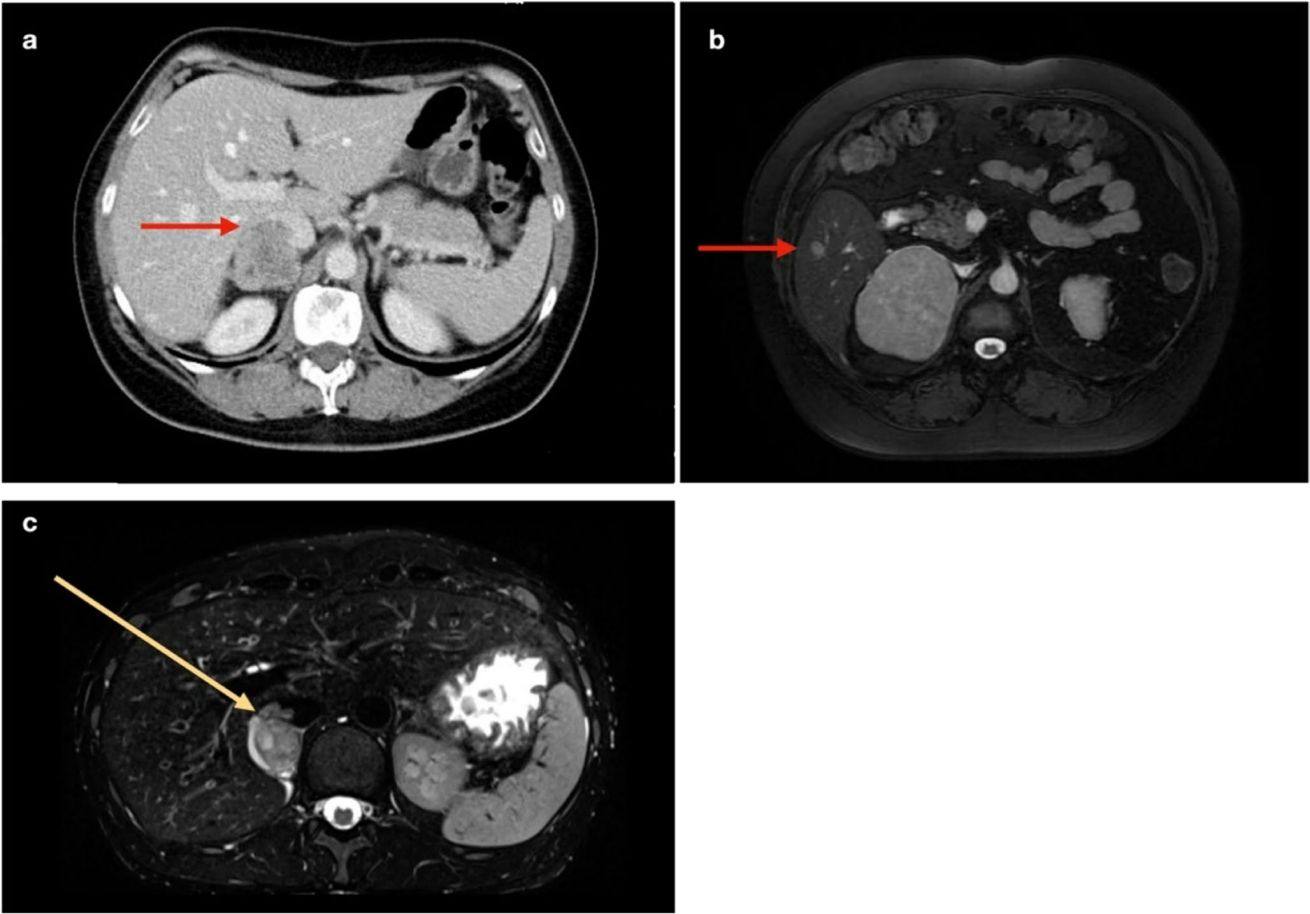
Preoperative imaging of patients with adrenal tumors clearly suspicious for ACC: a) computed tomography scan showing the invasion of the inferior vena cava (➔) b) magnet resonance imaging with an adrenocortical cancer and a concomitant solitary liver metastasis (➔) c) magnet resonance imaging showing ACC with a neoplastic thrombus in the IVC (➔)
